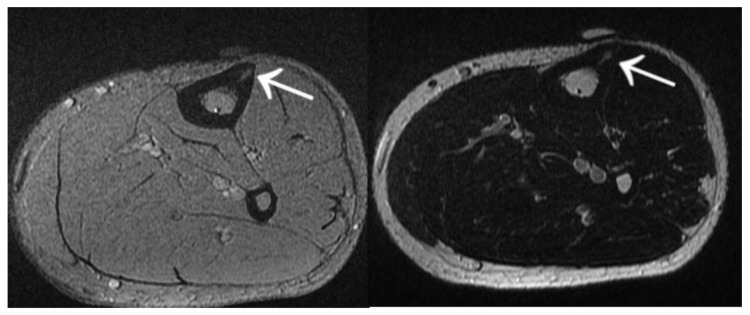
MRI scans showing a focus of OO (arrows) in the left tibia.
